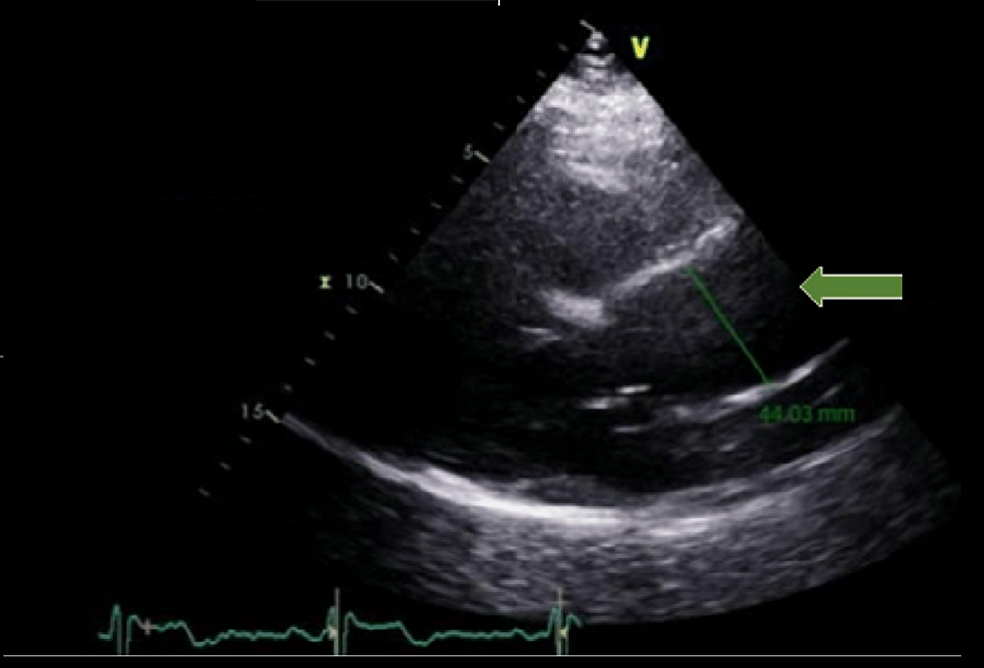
Parasternal long-axis view on transthoracic echocardiogram showing an ascending aortic dilation of 44 mm (green arrow)
